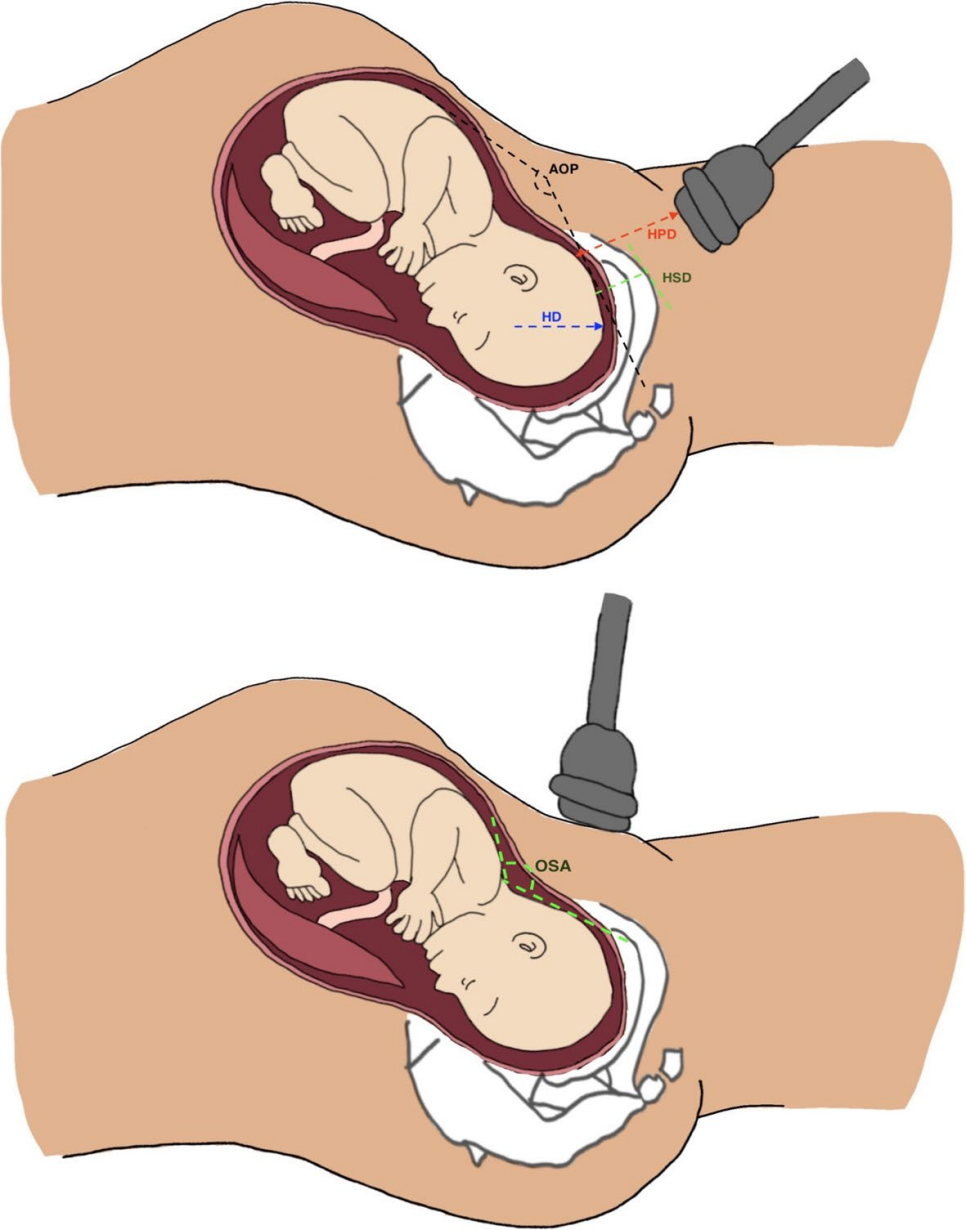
The OSA, HPD, AOP, HD and HSD of ultrasound parameters. OSA, occiput-spine angle; HPD, head-perineum distance; AOP, angle of progression; HD fetal head direction; HSD, head symphysis distance
